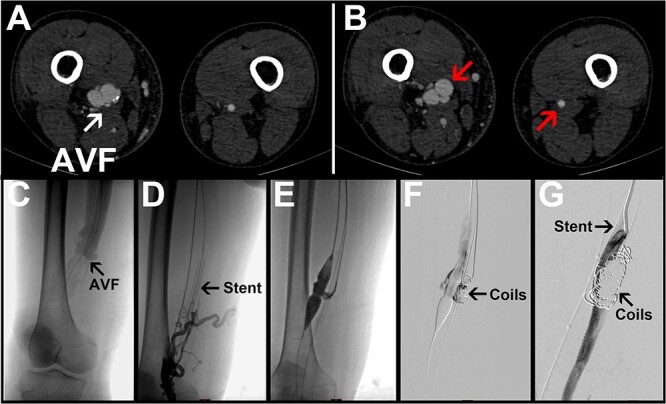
Representative images for Case 1. (A) Preoperative CTA image with the arrow pointing to an arteriovenous fistula. (B) The left arrow points to the obviously dilated right femoral artery; the right arrow points to the normal left femoral artery. (C–G) Intraoperative angiogram images. R, right side; L, left side; AVF, arteriovenous fistula.
